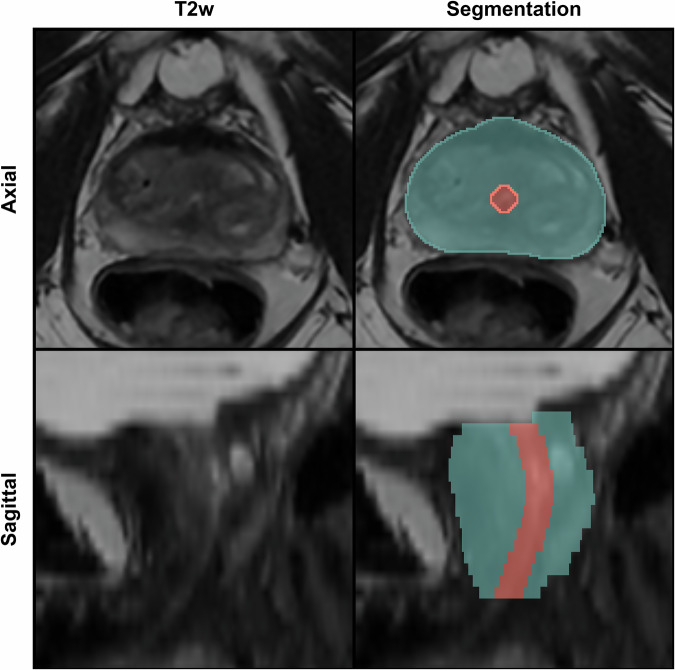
Example segmentation. An example segmentation of the prostate (light blue) and urethra (light red), displayed together with the corresponding axial T2-weighted image from the dataset.
